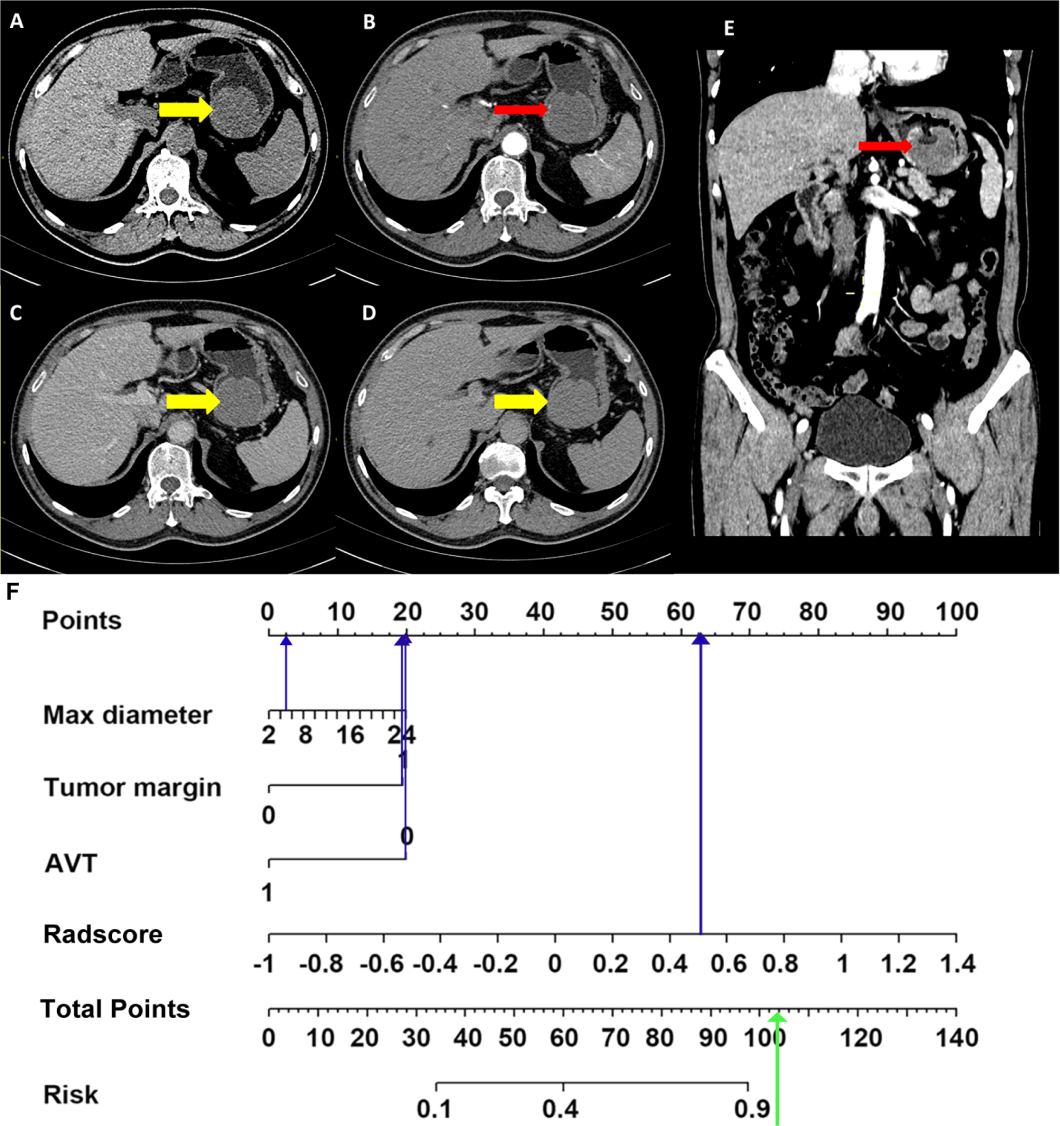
Application of combined model. Non-enhanced CT image (A), arterial phase images (B, transverse section; E, coronal section), portal venous phase image (C), delayed phase image (D), nomogram (F). A 58-year-old male was seen 1 week after the discovery of blood in the stool. Enhanced abdominal CT image shows a soft tissue density mass in the stomach (A, thick yellow arrows in CT images), with 5 cm diameter and unsmooth margin, no obvious enhanced vascular was seen in tumor (B, E, thin red arrows). The max diameter, tumor margin, AVT and Radscore points were substituted into the nomogram (blue arrow in Figure 7F) to obtain the total points. It was found that the probability of predicting KIT exon 11 deletion was greater than 90% (green arrow in Figure 7F). The pathological results of the patient showed KIT exon 11 deletion.
